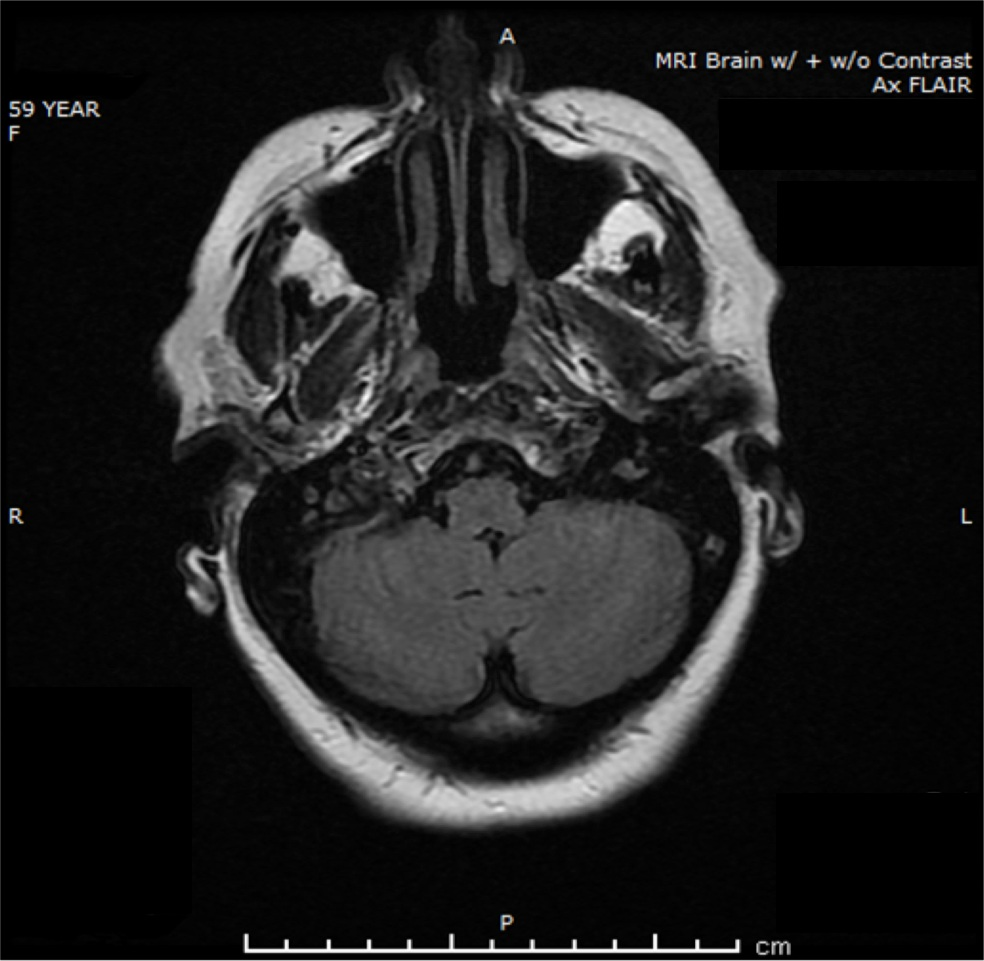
Magnetic resonance imaging one year ago, at the time of remission
